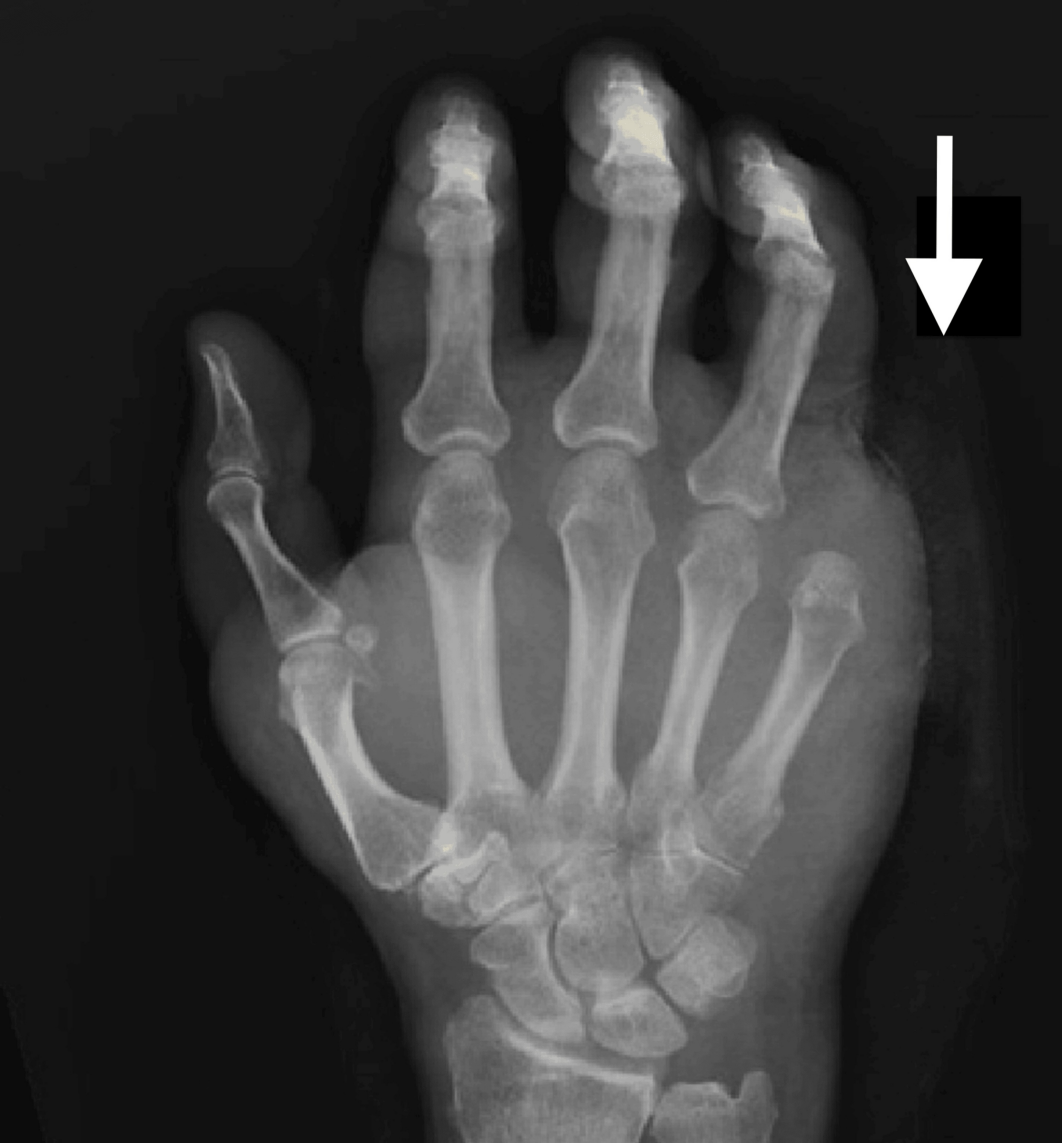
Postoperative X-ray imaging of the right hand following the amputation of the right fifth finger with disarticulation through the metacarpophalangeal joint. The arrow denotes the digit that was amputated.
